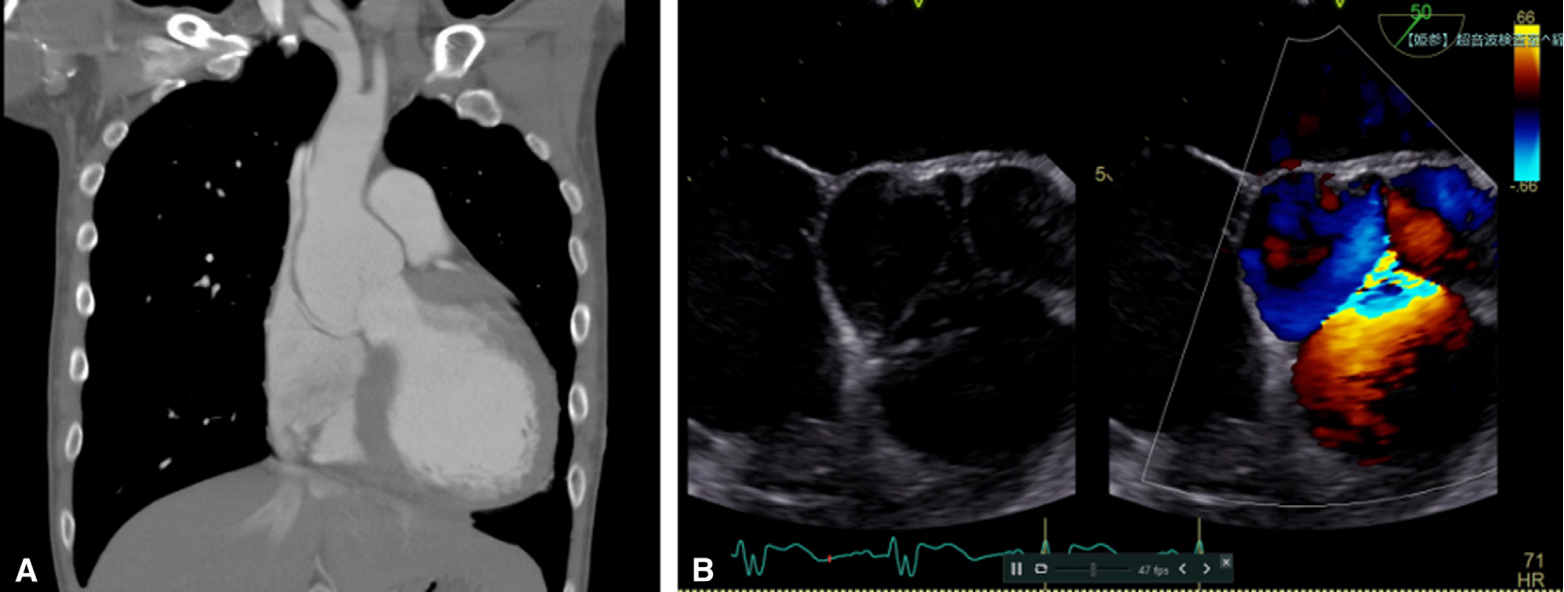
A, Preoperative computed tomography showed a root aneurysm. B, Preoperative transesophageal echocardiography showed severe aortic regurgitation and prolapse of the right coronary cusp with bending.
